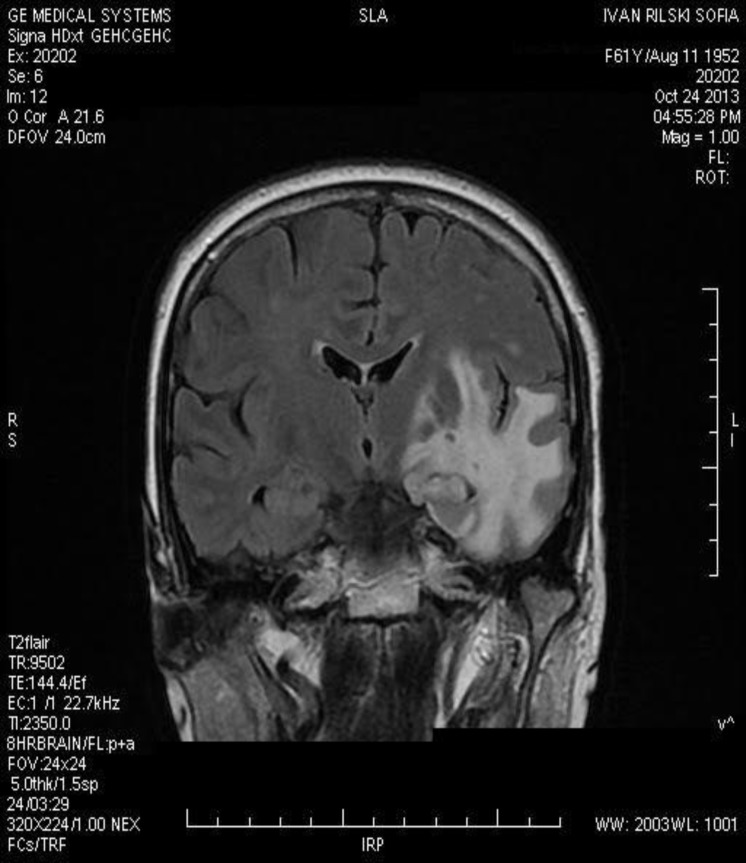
MRI image of the brain. It shows an infiltrative neoplastic lesion involving left temporal parenchyma
